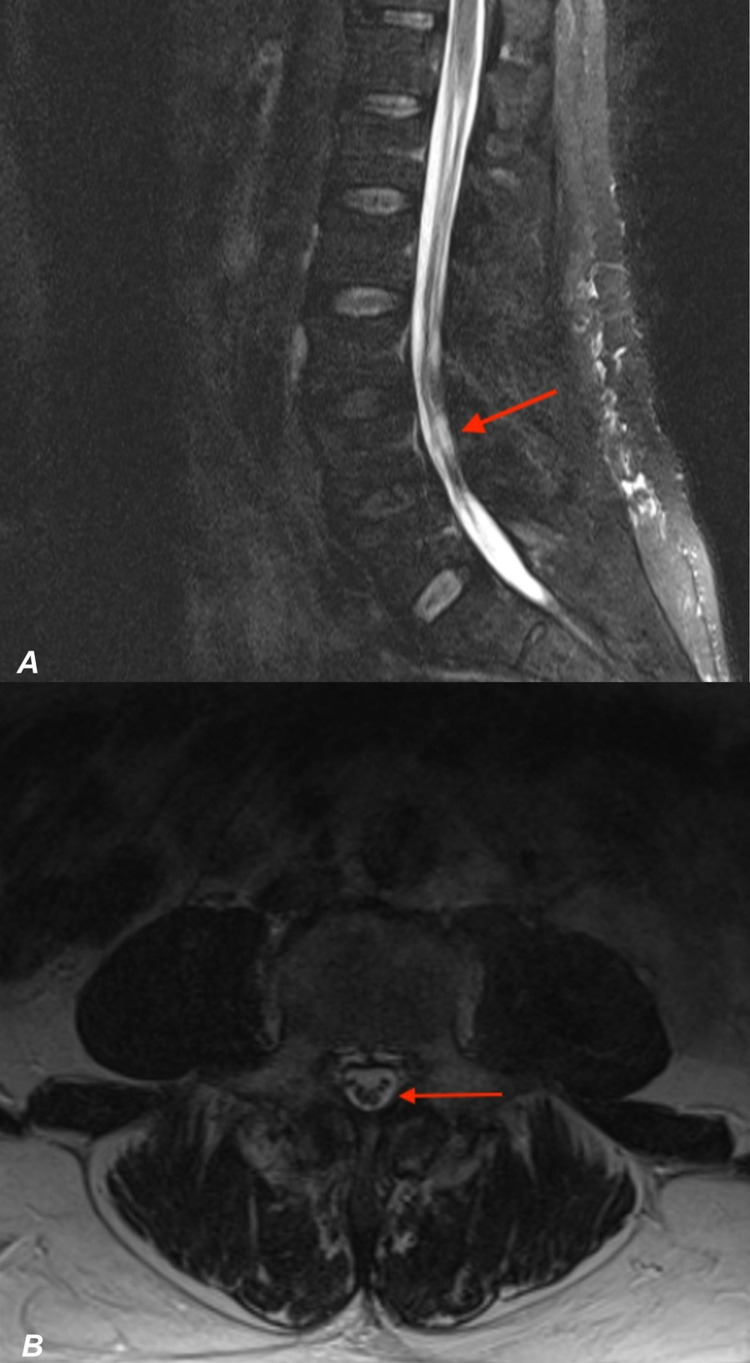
MRI of the spine shows inflammation of cauda equina Longitudinal (2A) and transverse (2B) T2 weighted MRI without contrast of the patient’s lumbar spine demonstrating thickening of the nerve roots of the cauda equina (arrows) and heterogenous appearance of cerebrospinal fluid (CSF) within lower lumbar spinal canal with minimal degenerative anterolisthesis at L4-L5.
